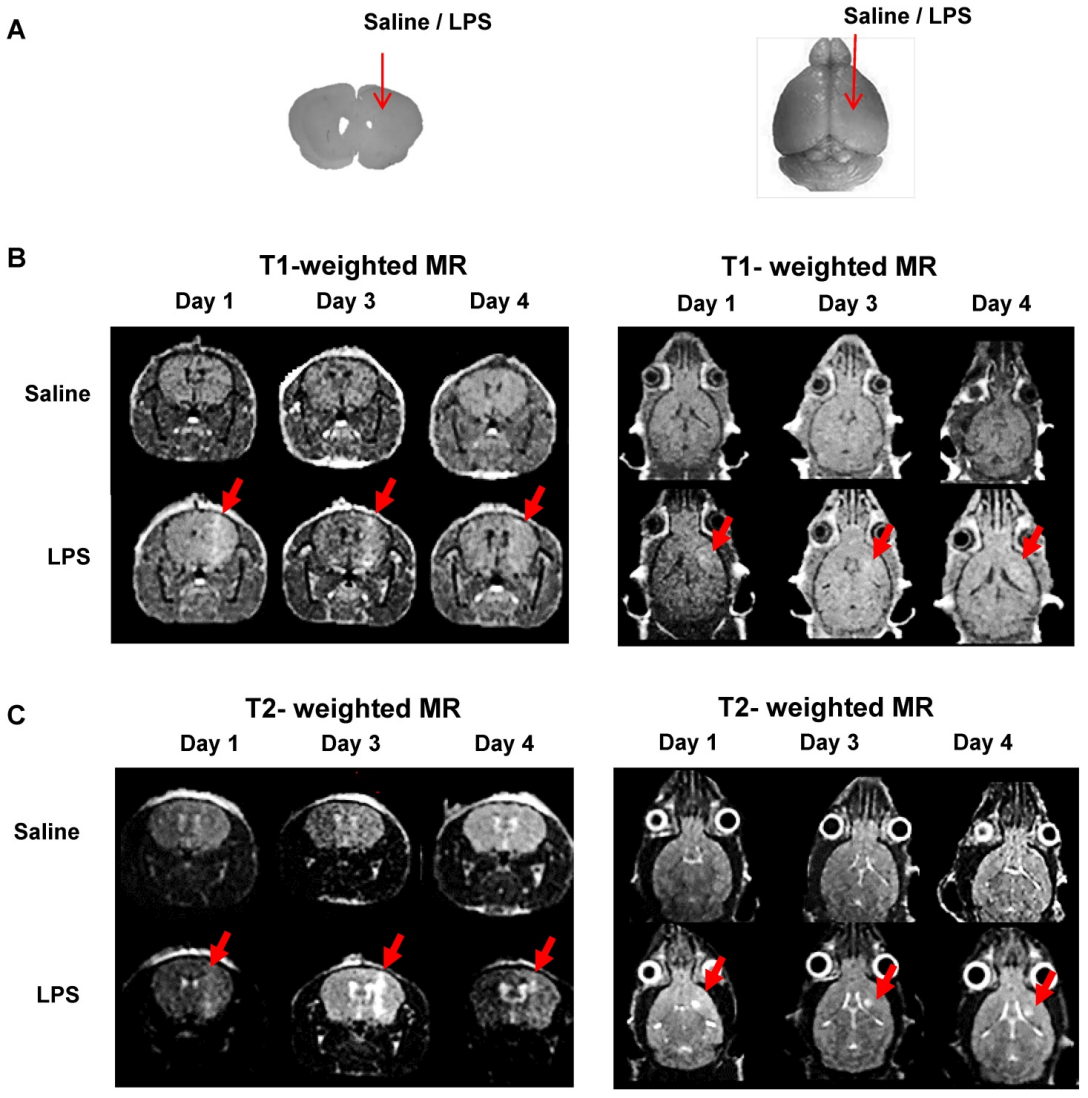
Representative MR scans using gadolinium-DOTA to monitor the Blood-Brain Barrier (BBB) disruption and immune cell activation. (A) Intracranial LPS injection model (saline as a control). (B) T1-weighted MR scans and (C) T2-weighted MR scans on day 1, 3, and 4 after intracranial injection (N=3). T1-weighted MRI was used to visualize the presence of gadolinium penetration through disrupted BBB, and T2-weighted MRI was used to monitor the vasogenic edema and localization of viable immune cells due to LPS induced-neuroinflammation.
